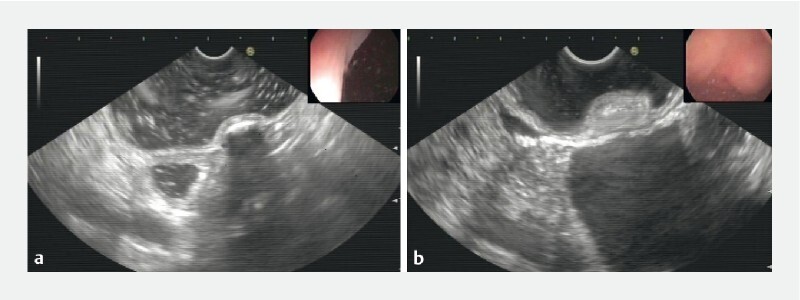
Endoscopic ultrasonography showed that the submucosal appendiceal lesion had clear boundaries with a section size of 14.3 × 8.9 mm, and the acoustic shadow was visible behind.
